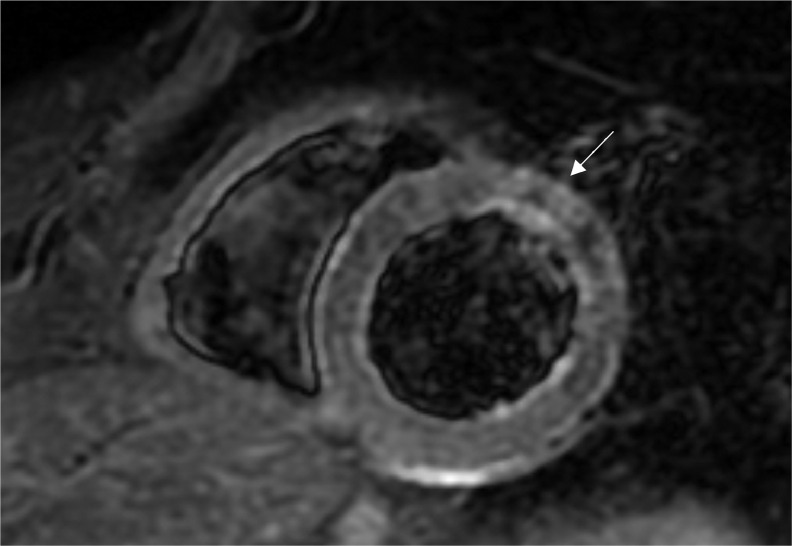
Cardiac magnetic resonance T2-weighted short axis view, showing focal area of edema involving the intramyocardial region of the middle segment of the anterior wall (white arrow).
